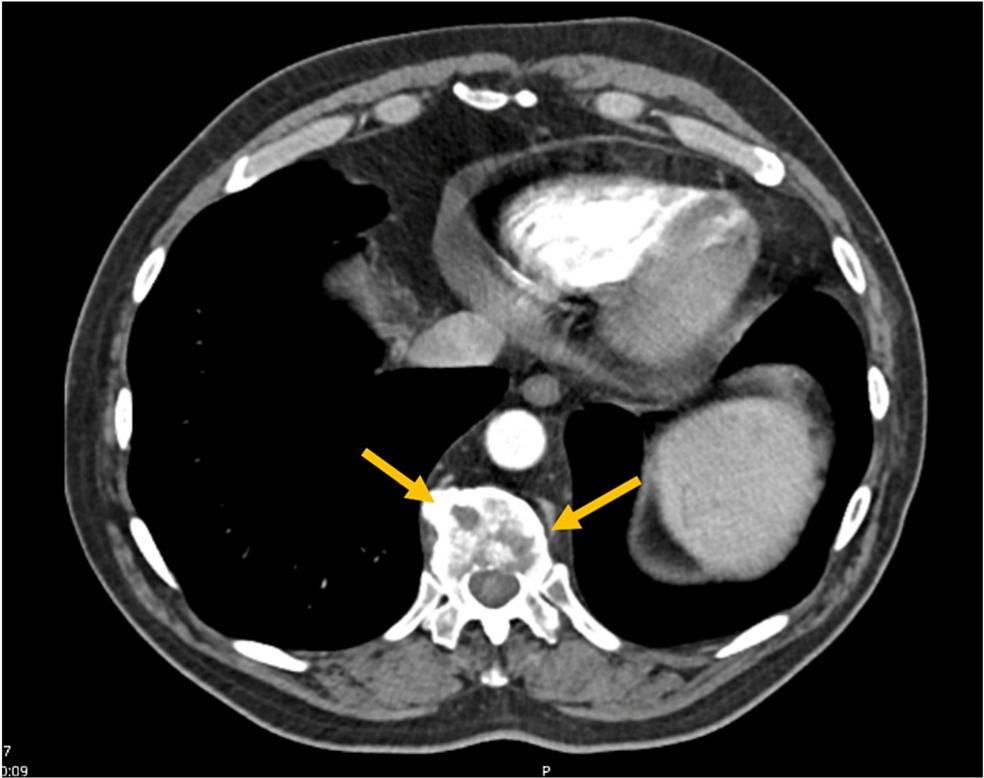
Thoracic CT scan showing vertebral lytic lesions
